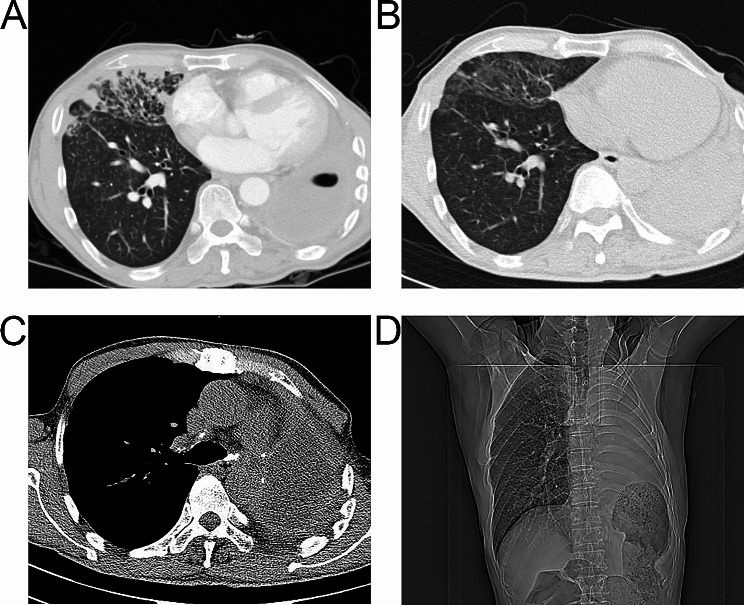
A-B. His right middle and lower pneumonia improved significantly compared with the previous period. No BPF could be seen on repeat chest CT within 3 months of postoperative follow-up. The residual cavity has been closed within 3 months of postoperative follow-up
